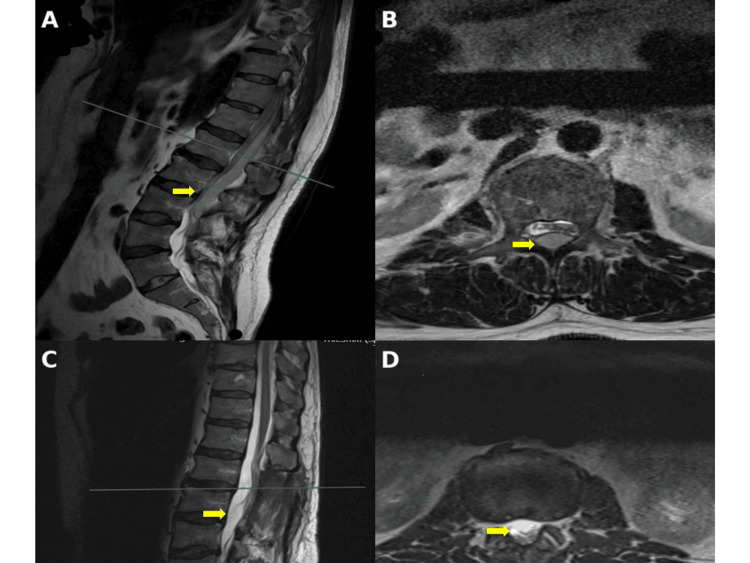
Preoperative and postoperative MRI findings in a 58-year-old male patient with extensive SEA (A) Preoperative sagittal MRI demonstrating a posterior epidural abscess extending across multiple spinal levels with significant spinal cord compression. (B) Preoperative axial MRI confirming circumferential epidural collection and canal compromise. (C) Postoperative sagittal MRI following limited decompression and catheter-directed epidural irrigation showing resolution of the epidural collection. (D) Postoperative axial MRI demonstrating adequate decompression of the spinal canal. SEA, spinal epidural abscess
